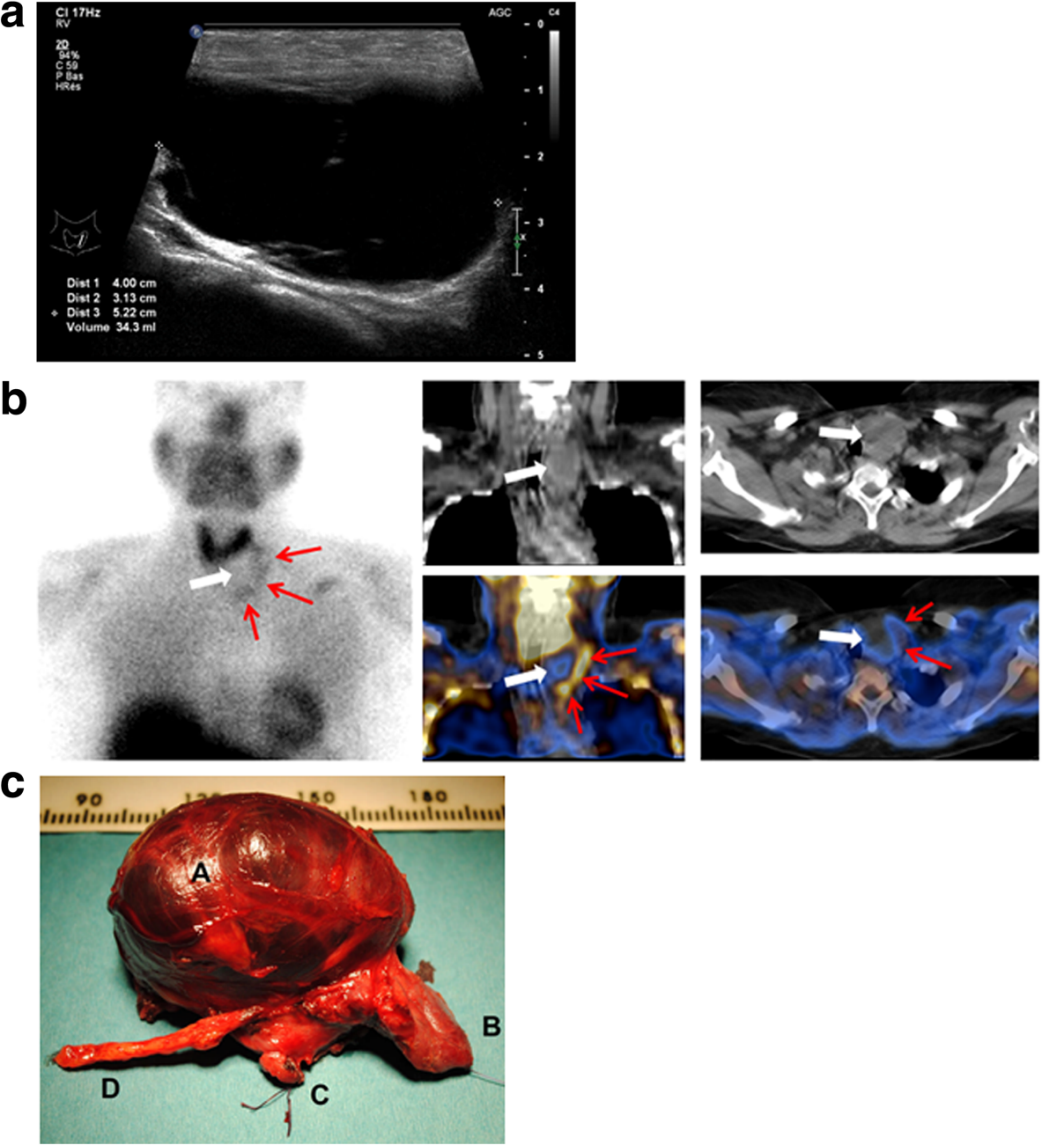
Patient 3. a Cervical ultrasound and longitudinal view of a large cyst. b Technetium 99 m sesta methoxyisobutylisonitrile scintigraphy with single-photon emission computed tomography-computed tomography. A central “cold” area (*white arrow*) corresponding to the parathyroid cyst is surrounded by faint tracer retention (*red arrows*) corresponding to the displaced parathyroid parenchyma. c Left thyroid lobe *en bloc* with the parathyroid cyst. *A* Cyst, *B* upper part of otherwise normal looking thyroid gland, *C* isthmus, *D* thymic remnant
